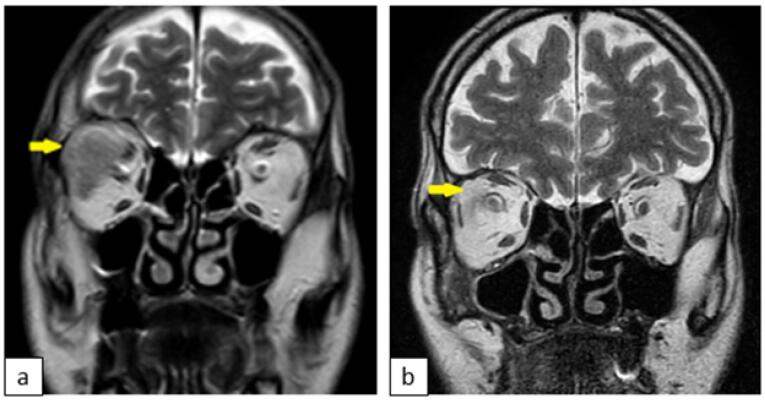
Renal mass biopsy of the lesion performed using a Tru-Cut 18-gauge needle under ultrasound guidance confirmed ChRCC (Figures 3a-3c).
